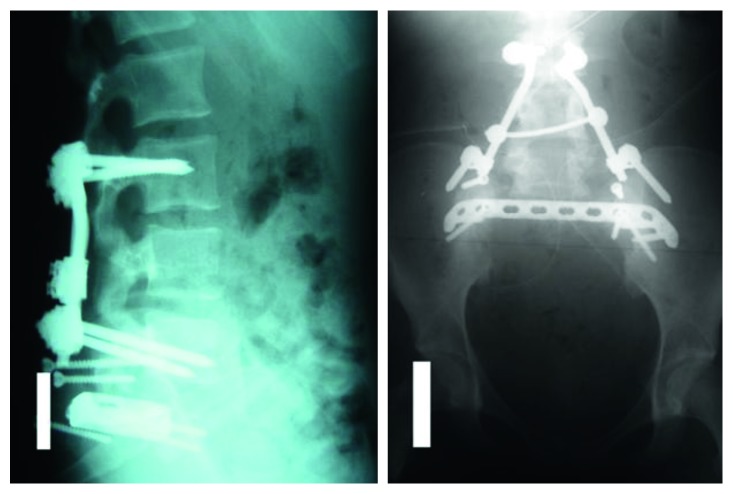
Postoperative X-rays of the lower spine in two axes (AP and lateral).
